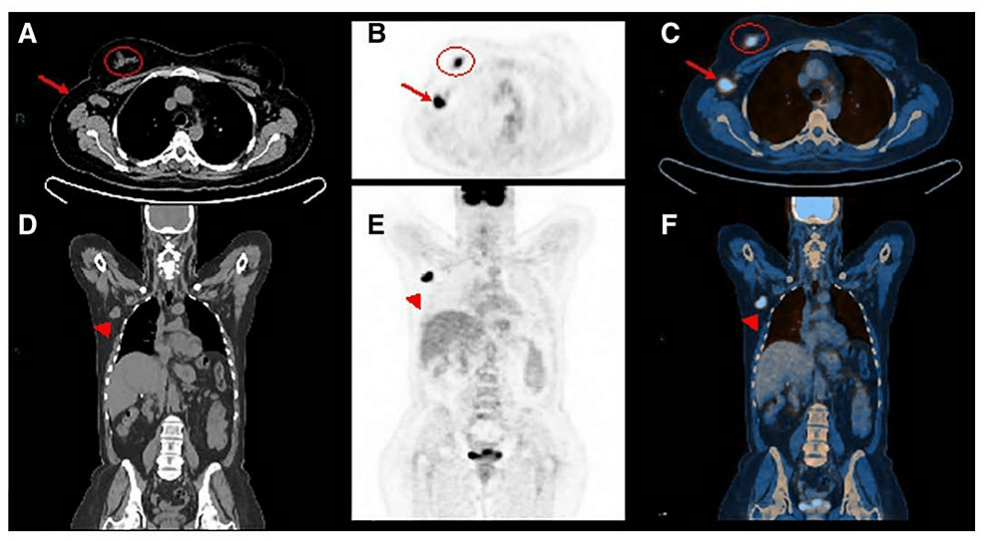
CT - based versus PET/CT based comparison of invasive ductal cancer of the right breast CT of the whole body: Axial (A) and coronal (D) views show enlarged node in the right axilla (A, arrow; C) associated with an irregular mass within the right breast (A, circle). PET of the whole body: Axial (B) and coronal (E) views demonstrate high flurodeoxyglucose uptake for both the mass and the enlarged node in the axilla that is also confirmed by hybrid imaging PET/CT (C, F) CT: computed tomography, PET: positron emission tomography, PET/CT: positron emission tomography/computed tomography This image is reproduced from Marino et al. [56] - available via Creative Commons CC-BY-NC-ND license
